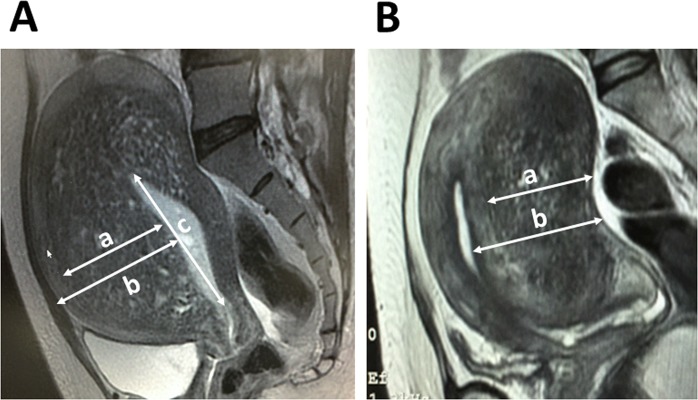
Subtype I and subtype II adenomyosis. T2-weighted magnetic resonance image (sagittal section) of subtype I (intrinsic, (A) adenomyosis and subtype II (extrinsic, (B) adenomyosis. a, Maximum diameter of adenomyosis-associated lesion with punctate high-intensity myometrial foci (adenomyosis thickness); b, maximum diameter of myometrial wall thickness (myometrial thickness); and c, maximum distance between the uppermost part of the uterine cavity and internal os (cavity longitudinal distance).
